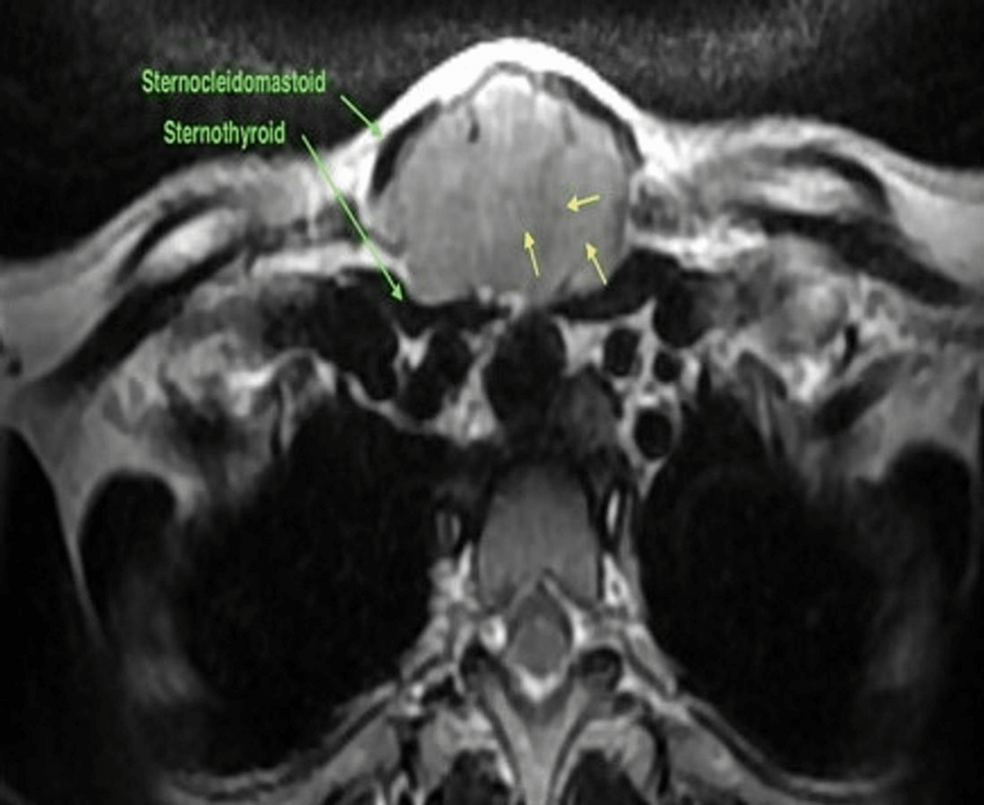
MRI neck (plain and contrast) suggestive of peripheral nerve sheath tumour. Schwannoma in the suprasternal region with yellow arrows showing fascicular sign
